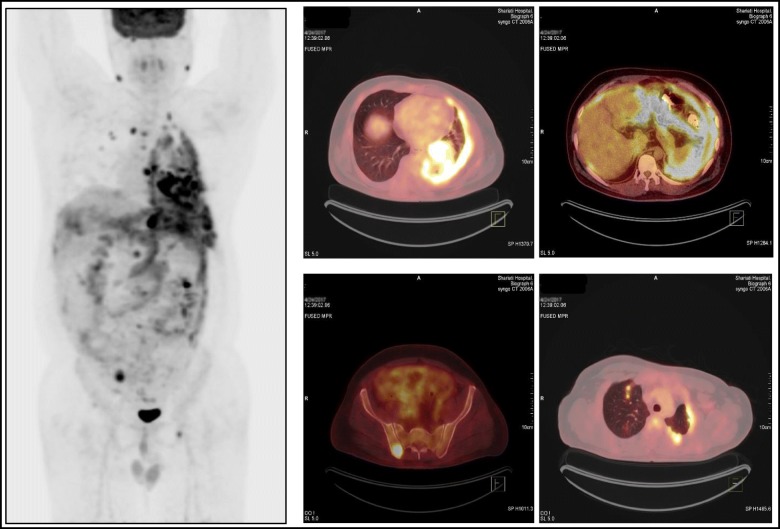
Imaging findings on fluorodeoxyglucose positron emission tomography/computed tomography scan
